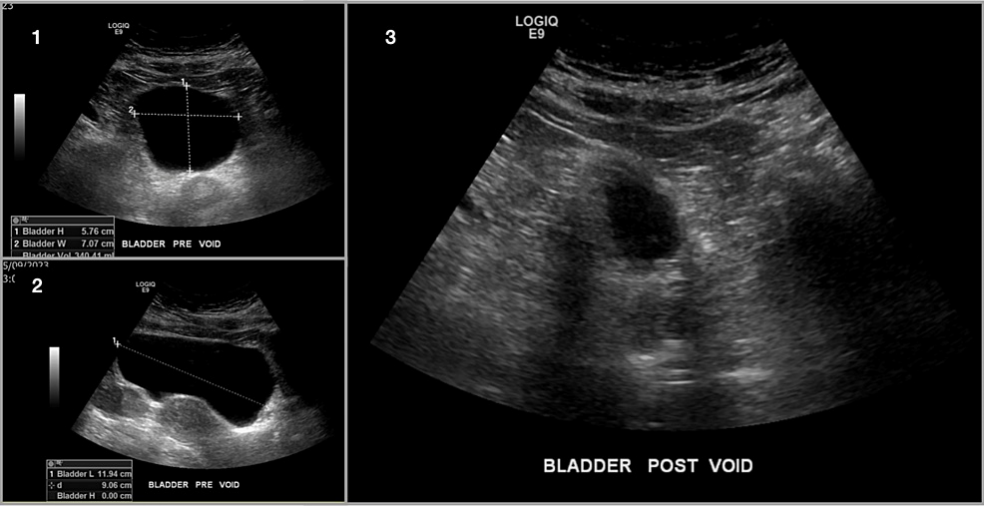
Bladder Ultrasound. 1- Bladder Pre-Void Sagittal View 2- Bladder Pre-Void Transverse View 3- Bladder Post-Void
